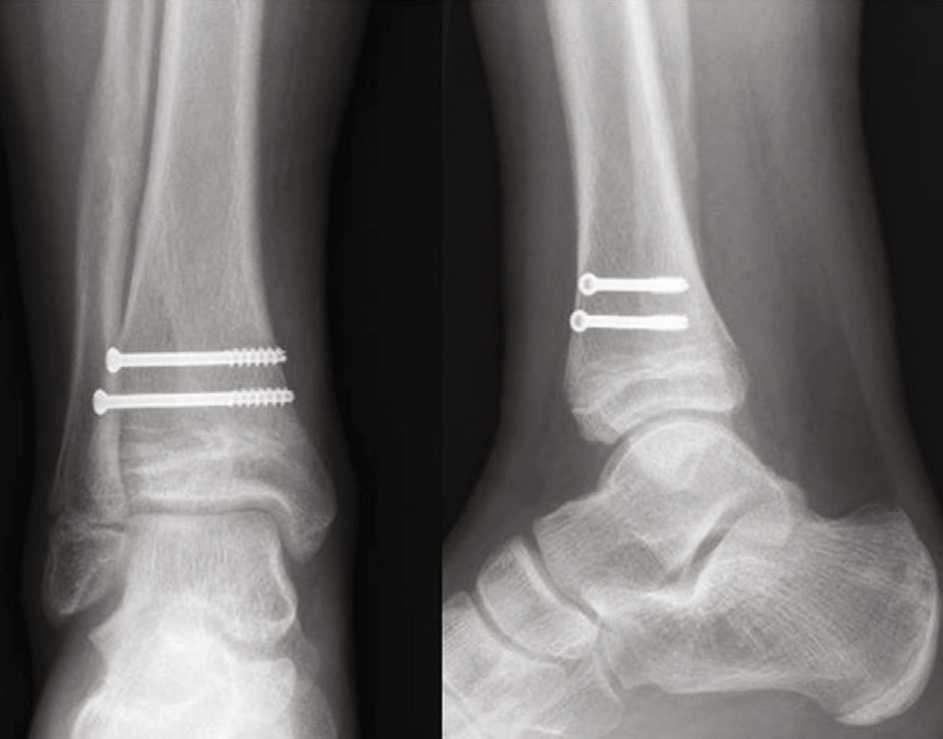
Anteroposterior and lateral radiographs of the ankle at 18-month follow-up.
